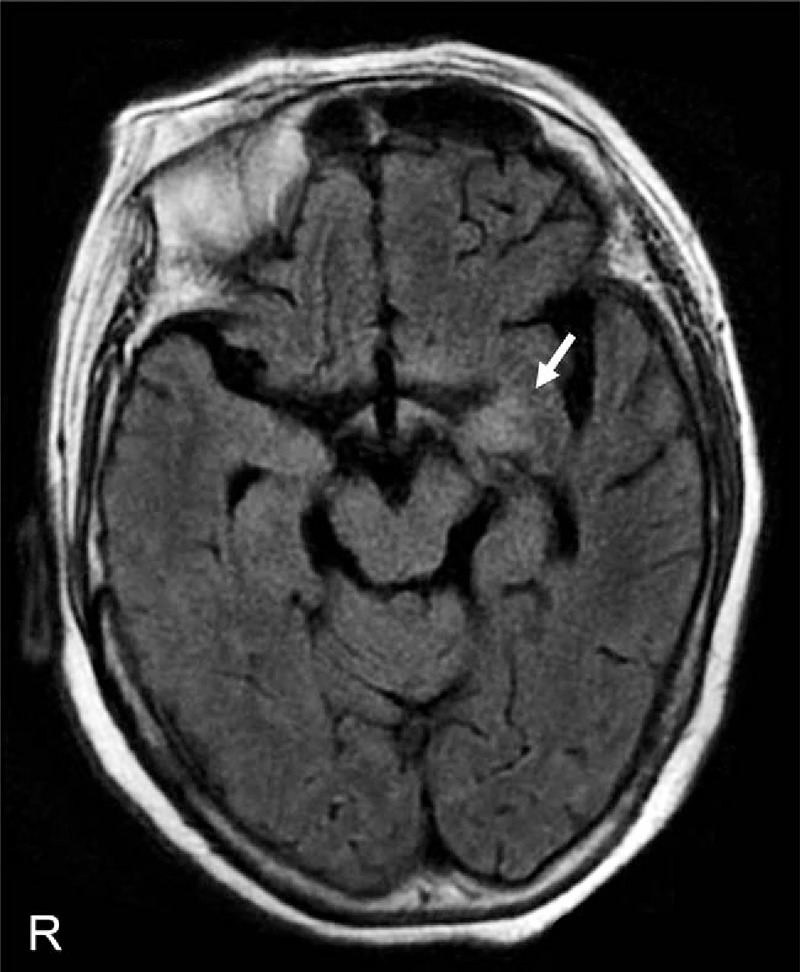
Brain MRI findings of a representative patient (Case 12). Fluid-attenuated inversion recovery images show abnormal hyperintense signals in the left medial temporal lobe (arrow). MRI = magnetic resonance imaging.
